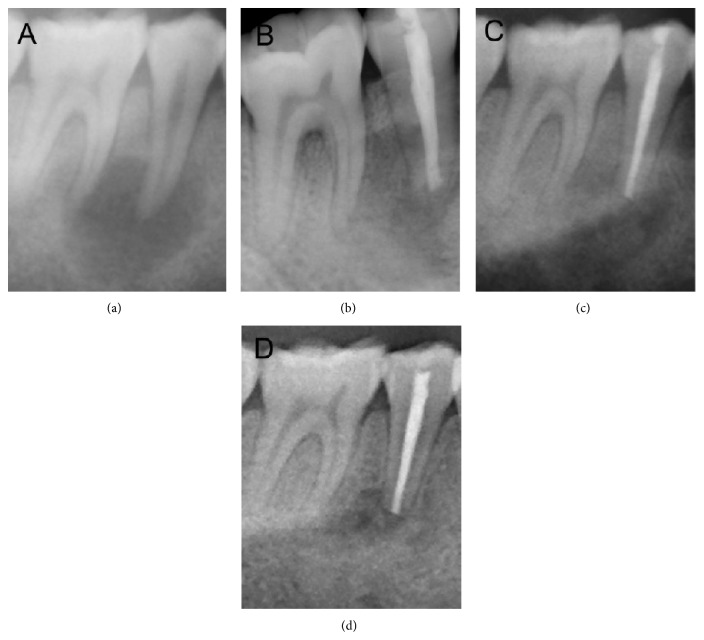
Radiographs. (a) Radiograph at the initial visit showing a large periapical lesion associated with tooth 45, measuring 14 × 15 mm in diameter. (b) A periapical radiograph of tooth 29 and tooth 30 to observe and record the immediate postoperative situation. (c) Radiograph after 3 months showing a decrease in the size of the periapical radiolucency to 13 × 14 mm in diameter and some material implanted seemed to be absorbed. (d) Radiograph after 7 months showing an apparent decrease in the size of the radiolucent area to 4 × 5 mm in diameter.
